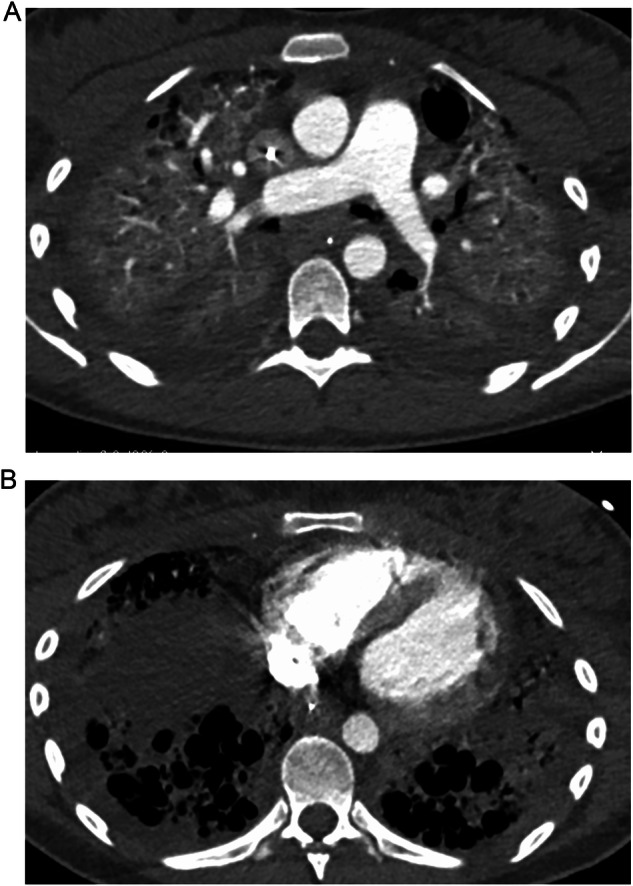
A, B CT scans of a patient with drug-induced interstitial lung disease showing the acute interstitial pneumonia / acute respiratory distress syndrome pattern, including bilateral consolidations and pleural effusion (diagnosed by multidisciplinary team consensus)
